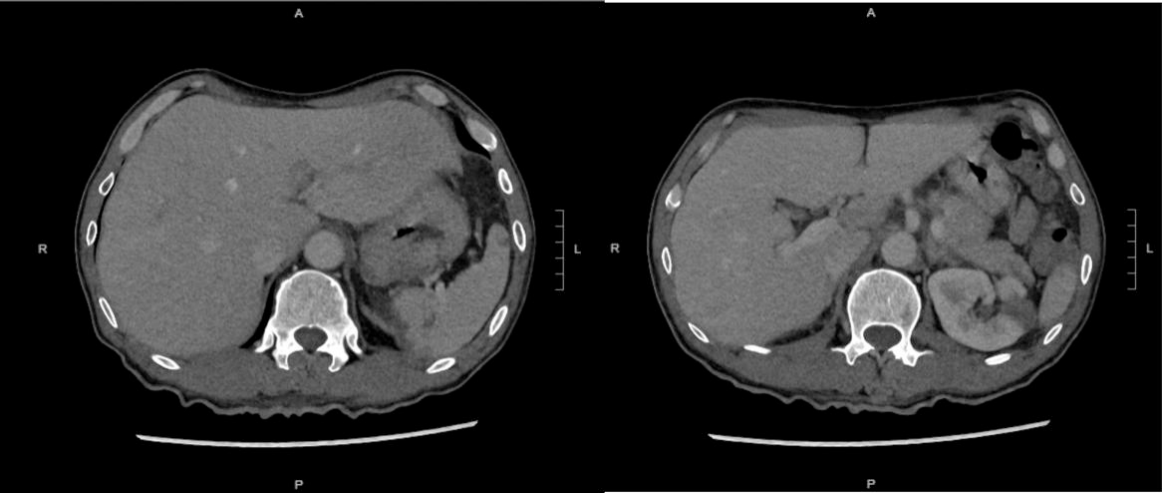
CT of the abdomen and pelvis showed splenic and renal infarcts.
